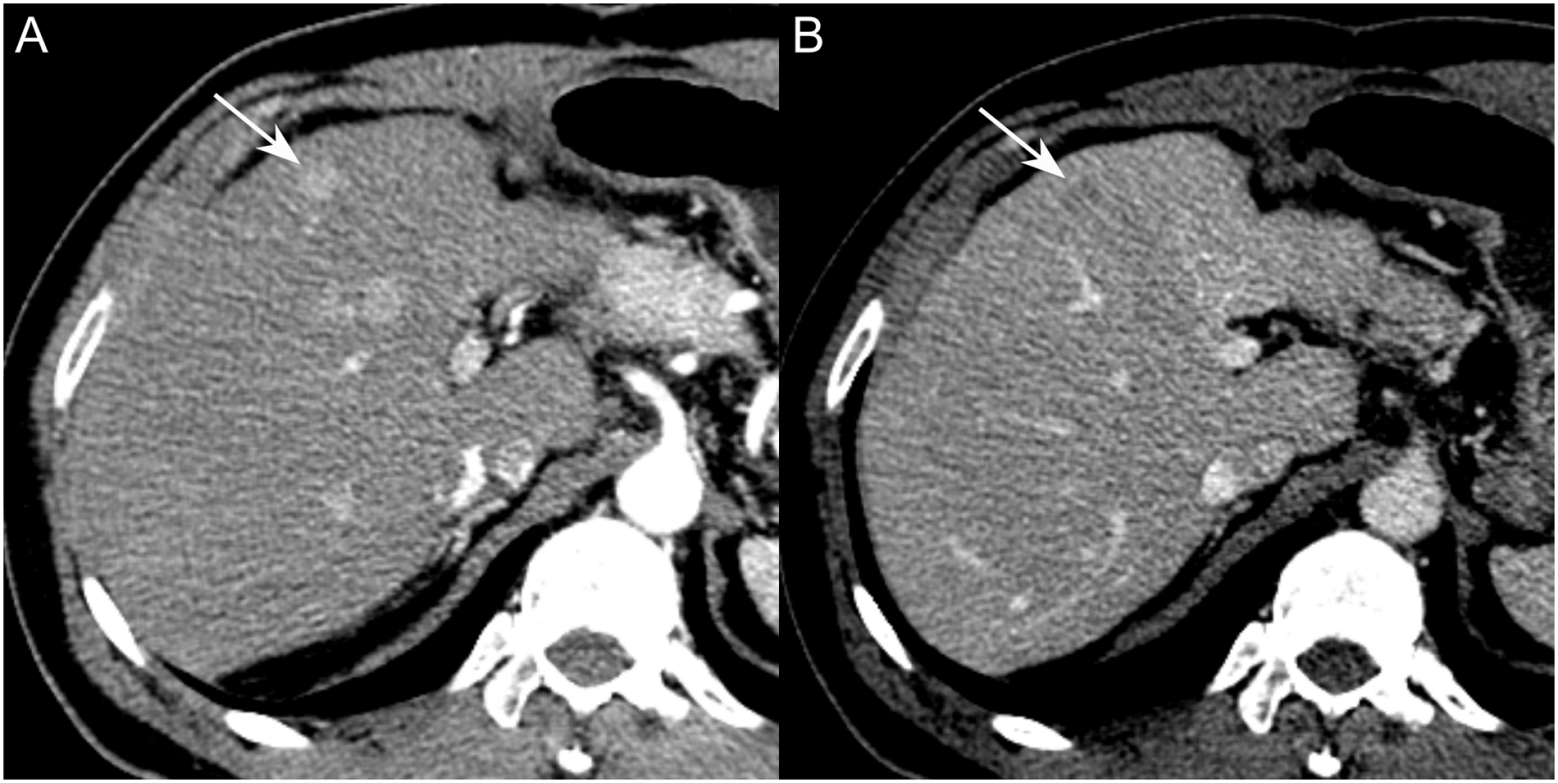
58-year-old man with type I HCC larger than 1 cm in diameter. A-B, On the contrast-enhanced CT in arterial (A) and portal venous (B) phases images, a 14 mm sized nodule in S4 of the liver showed arterial enhancement and washout (type I). This lesion was reported as HCC in the formal report, and both reviewers also assessed this lesion as HCC. After TACE, compact nodular uptake of Lipiodol was seen on the post-TACE and follow-up CT (not shown).
